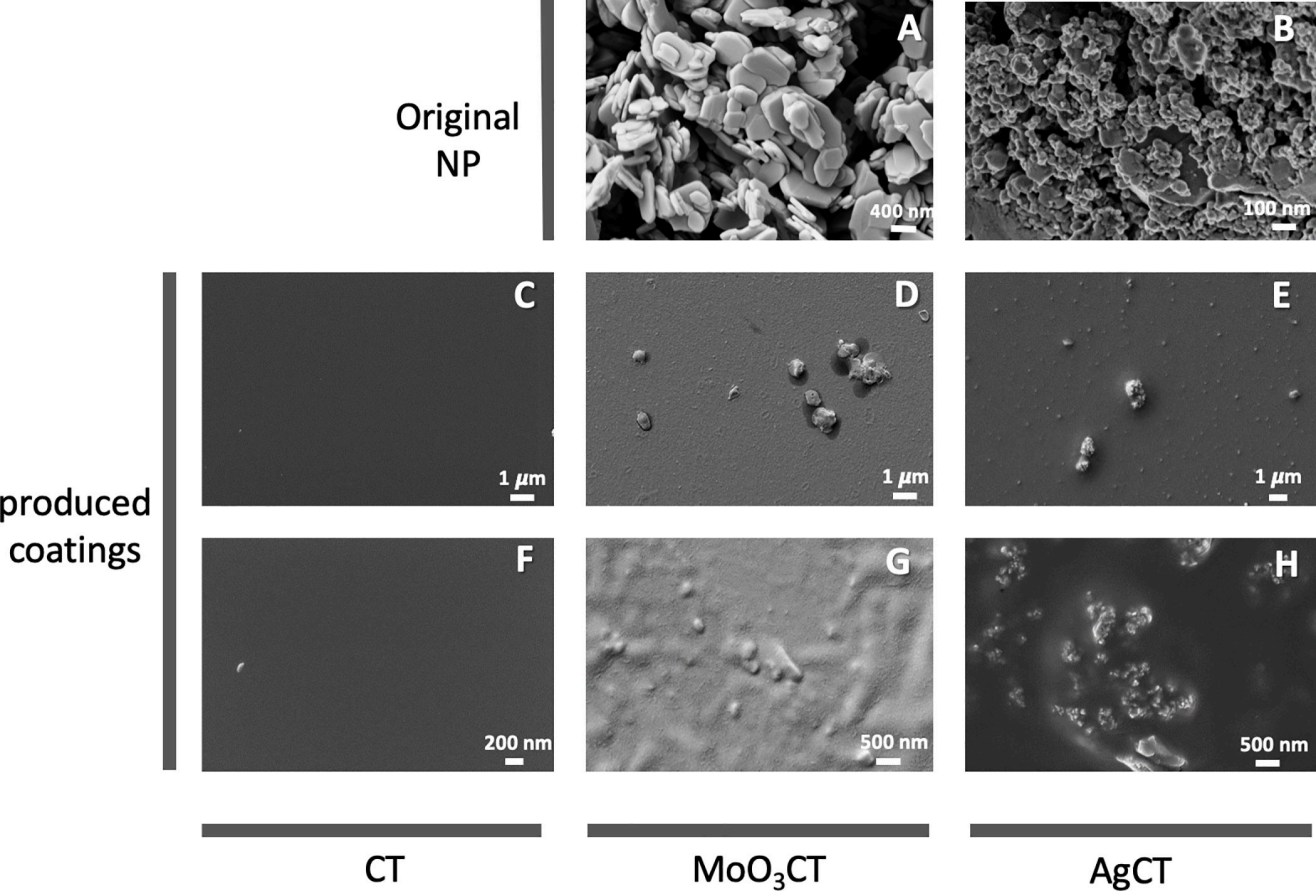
SEM-FIB images of the MoO_3_ and Ag NP (A and B, respectively) and the produced coatings; CT (C and F), MoO_3_CT (D and G), and AgCT (E and H).
